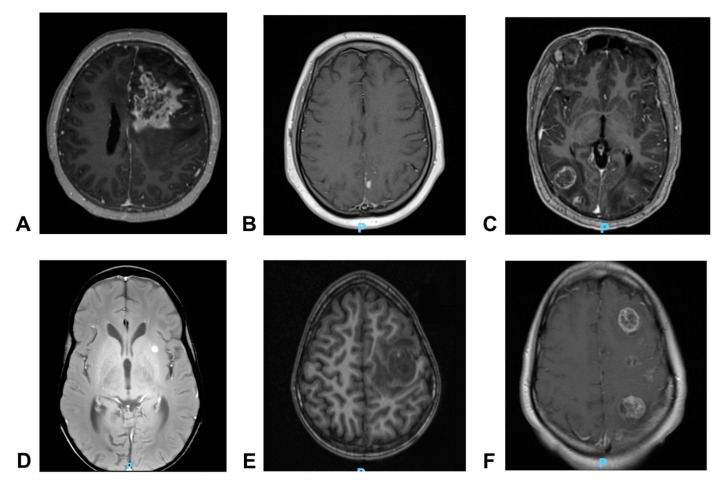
Representative axial T1 MRI imaging initial brain metastases of (A) Patient 1; (B) Patient 2; (C) Patient 3; (D) Patient 4; (E) Patient 5; and (F) Patient 6.
